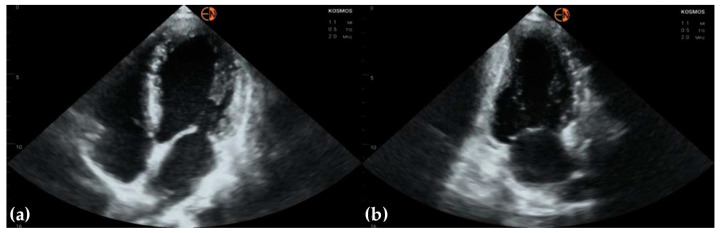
(a) A4C and (b) A2C view on a patient presenting with peripheral arteriopathy (angioplasty of left superficial femoral artery). The mitral valve was classified as having no regurgitation on handheld examination, while conventional ultrasound showed mild mitral regurgitation.
